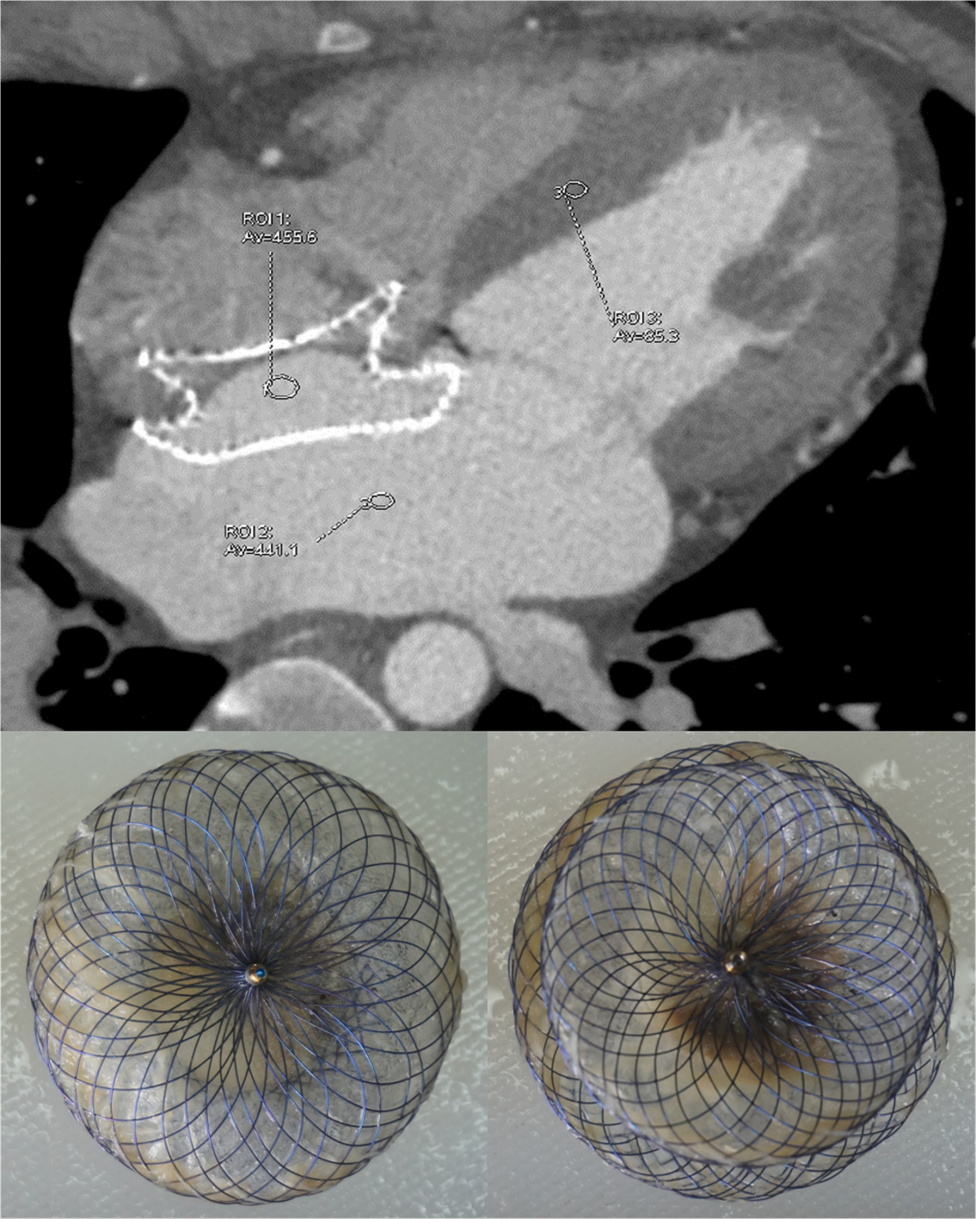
Complete contrast uptake within the occluder on cardiac computed tomography angiography (CTA). Cardiac CTA demonstrated complete contrast uptake within the occluder in a patient with an implantation period of 4 years (upper panel). On either disc, there was almost no endothelialization with complete exposure of device struts (lower). *Note*: *local CT density was 455*.*6 HU within the device*, *85*.*3 HU in the myocardium*, *and 441*.*1 HU in the left atrium; ROI*, *region of interest; Av*, *average CT density; CT*, *computed tomography; HU*, *Hounsfield units*.
